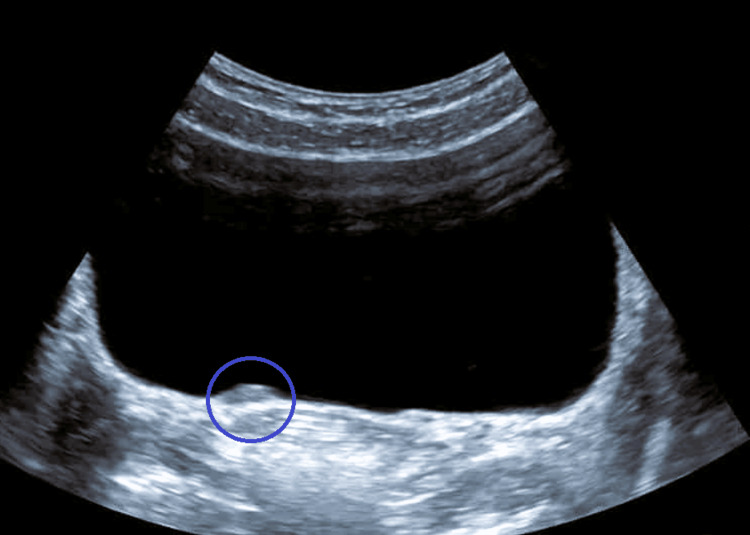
Ultrasound image of the urinary bladder of a patient with NA. Hyperechoic thickening about 10 mm in diameter marked by a circle on the right posterolateral wall of the urinary bladder NA: nephrogenic adenoma
